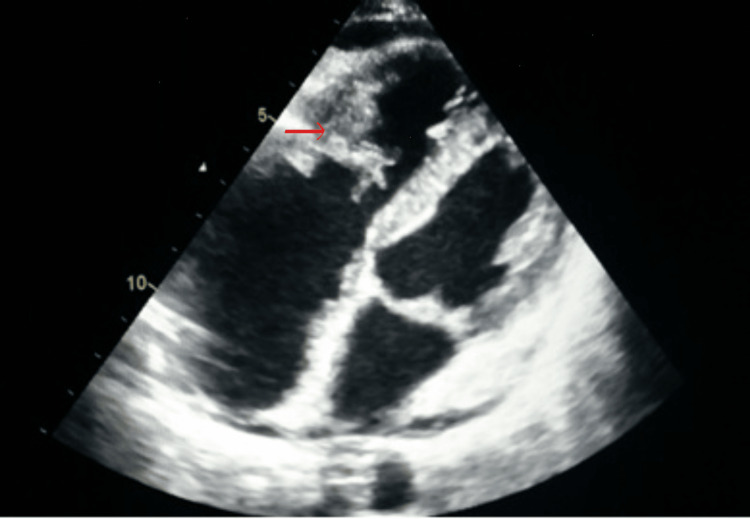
Four-cavity echocardiographic image showing nodular formations infiltrating the tricuspid valve and the RA. RA, right atrium
